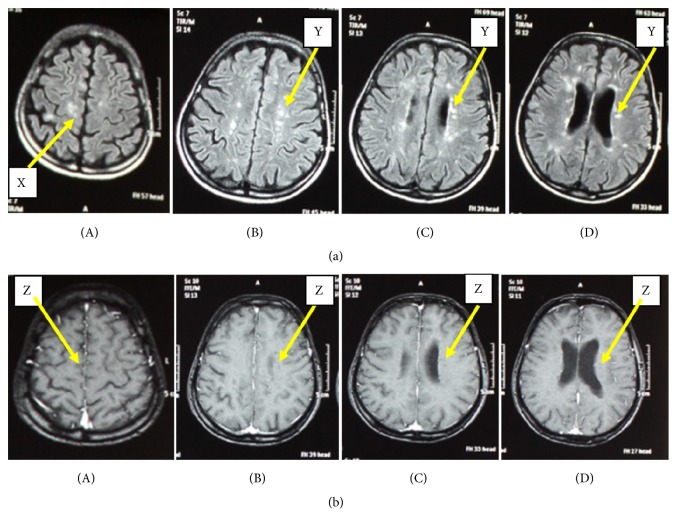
FLAIR hyperintense and T1W hypointense lesions in cerebrum of an MS patient*. (a) MRIs of McDonald criteria fulfilling MS patient showing sections of cerebrum with hyperintense lesions in FLAIR sequence; (a)(A) oval and round shaped subcortical lesions indicated by “X”; (a)(B), (a)(C), and (a)(D) oval periventricular lesions located perpendicular to the ventricular surface indicated by “Y” (Dawson's fingers appearance). (b) MRIs of a patient showing sections of cerebrum with hypointense lesions in T1W sequence; (b)(A) hypointense lesions in subcortical region indicated by “Z”; (b)(B), (b)(C), and (b)(D) hypointense lesions in periventricular regions indicated by “Z”.
